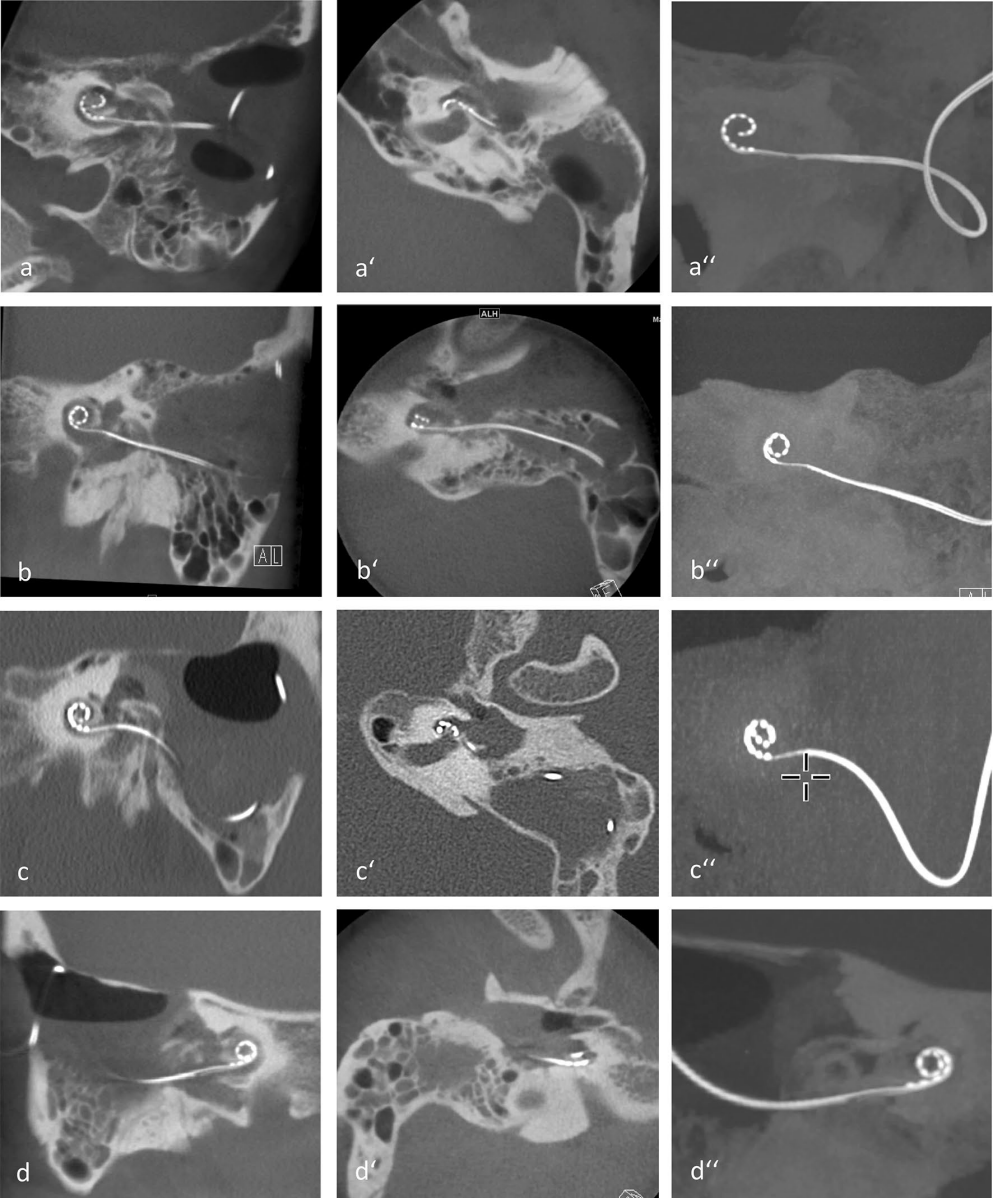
Postoperative Cone Beam Computed Tomography (CT) scans (apart from third row: high-resolution temporal bone CT) showing the electrode arrays in their final position with the remaining parts of the cochlea.  a–d Paracoronal multi-planar reconstructions (MPR),  a'–d' axial MPRs, a”–d” paracoronal maximum intensity projection (MIP), a–a” patient ID 1, b–b”  patient ID 2, c–c” patient ID 3, d-d” patient ID 4 from Table 1
